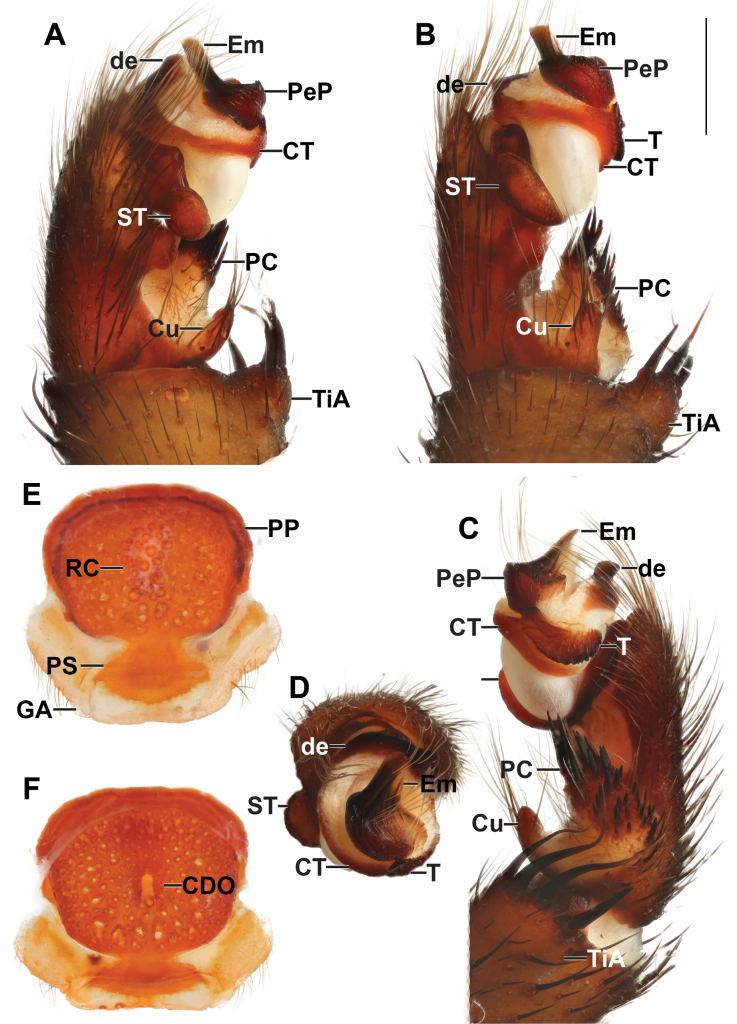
Liphistiusdawei* sp. nov. male palp and vulva plate A–D ARA-2018-143 (holotype) palp A prolateral view B ventral view C retrolateral view D distal view E, F ARA-2018-138 (allotype) vulva plate E ventral view F dorsal view. Abbreviations: CDO = central dorsal opening; CT = contrategulum; Cu = cumulus; de = distal edge of the contrategulum; Em = embolus; GA = genital atrium; mm = millimeter; PC = paracymbium; PeP = paraembolic plate; PP = poreplate; PS = posterior stalk; RC = receptacular cluster; ST = subtegulum; T = tegulum; TiA = tibial apophysis. Scale bar: 1 mm.
